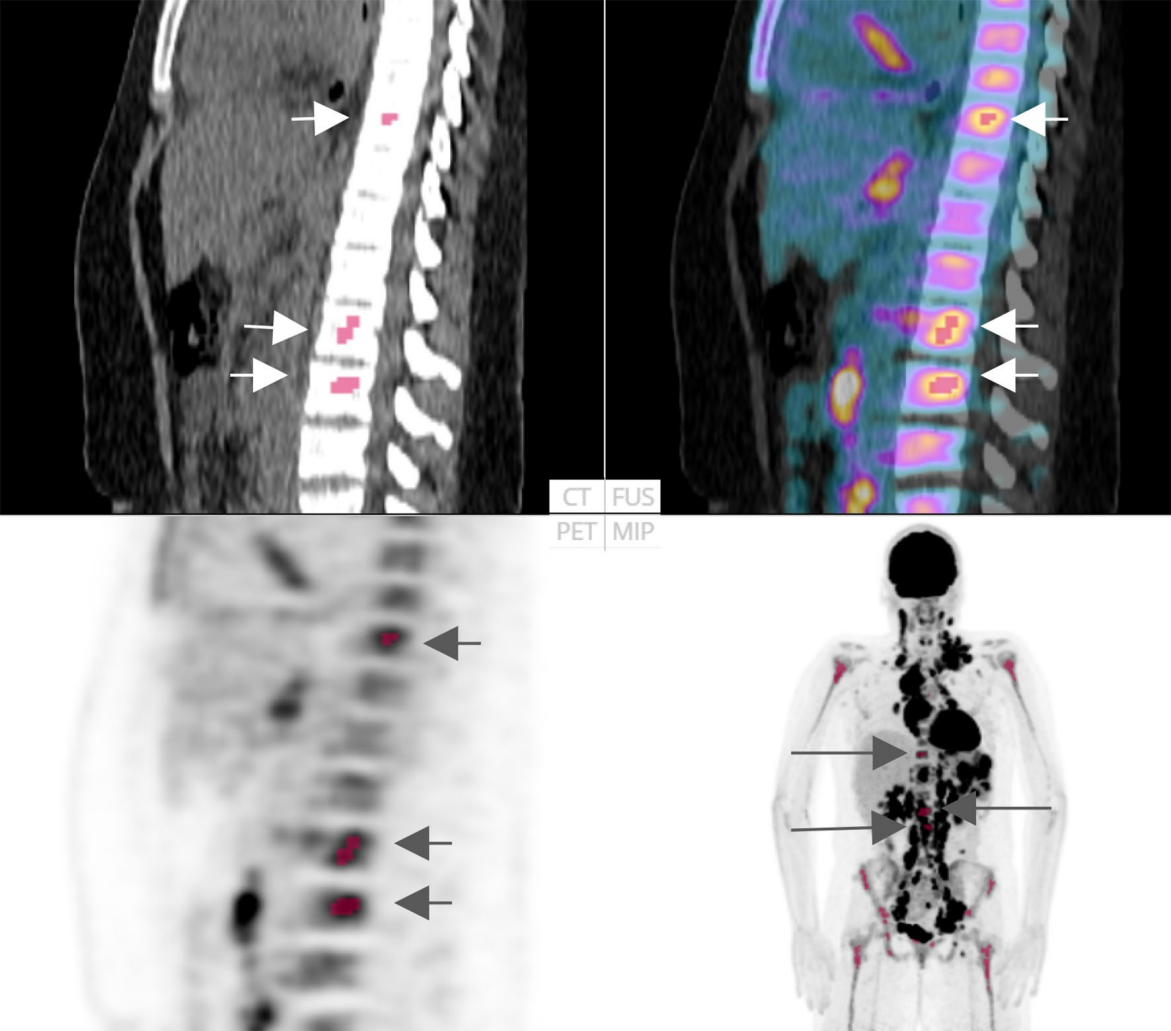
FDG-PET/CT: Example of a patient classified as having focal uptake in skeleton/bone marrow by the artificial intelligence-based method (highlighted in red and by arrows) and by the majority (8/10) of the physicians.
